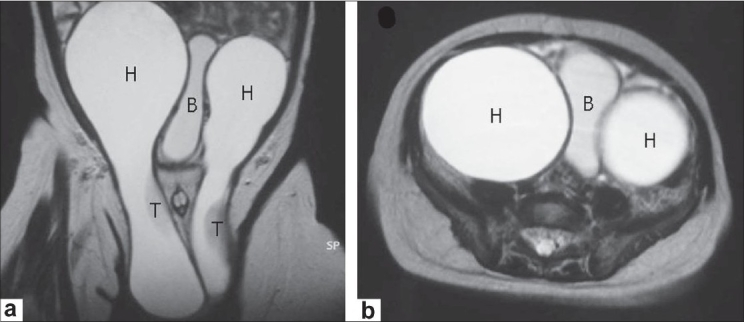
T2-weighted magnetic resonance images from the coronal; (a) axial; (b) views. The bilateral massive hydroceles extended into the abdomen through the inguinal canal. Hydrocele; H, Bladder; B, Testis; T
